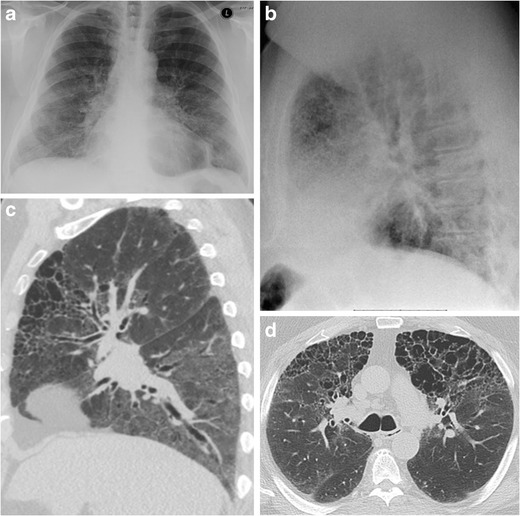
55-year-old man with smoking history. The frontal radiograph (a) is normal, and the lateral radiograph (b) shows reticular opacities in the retrosternal clear space only. Axial (c) and sagittal (d) CT slices show paraseptal and centrilobular emphysema corresponding to the radiographic abnormality. Ground-glass opacities in the lower lobes are suspected to be due to desquamative interstitial pneumonia
